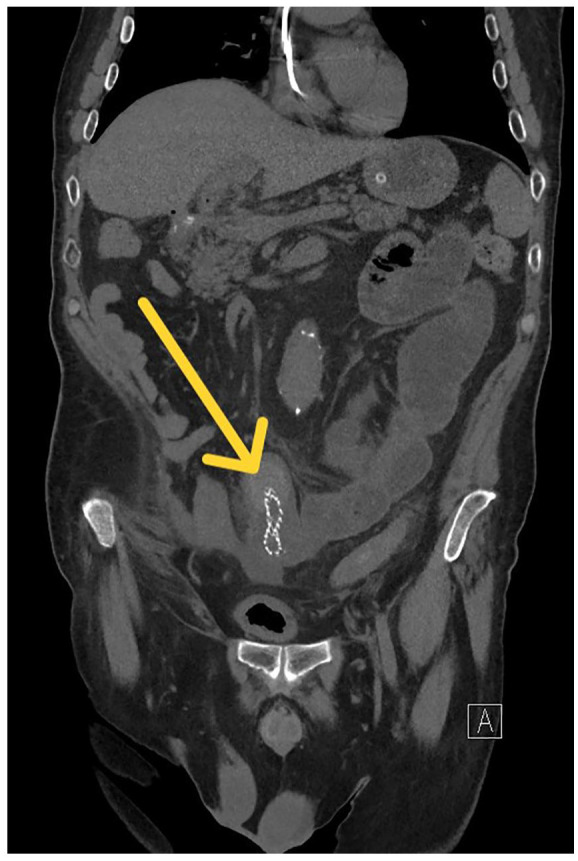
CT scan revealing small bowel obstruction and the migrated stent (arrow).
